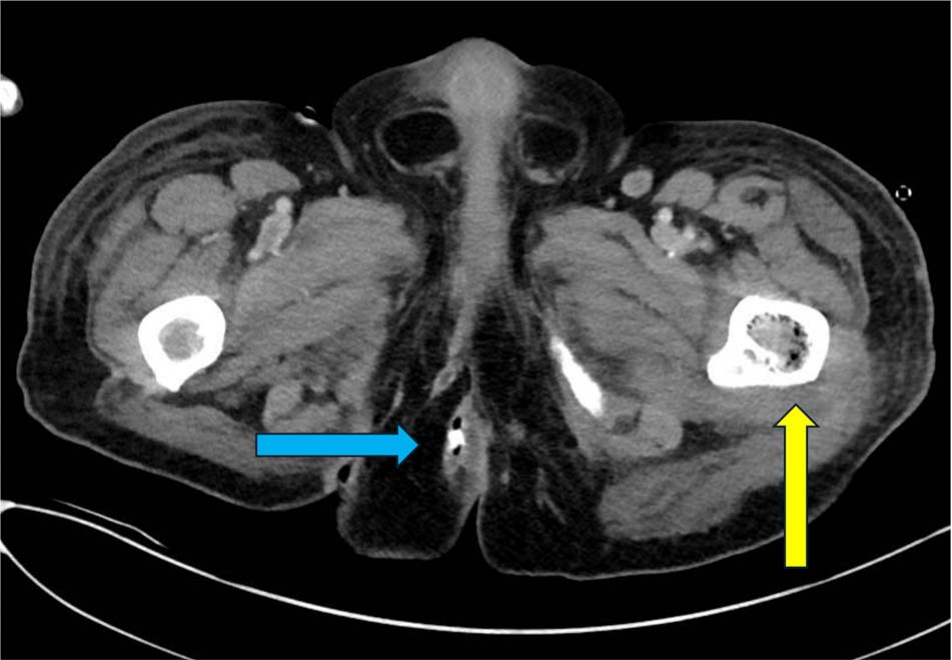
CT abdomen and pelvis—axial plane. Intraosseous gas is seen in the left femur at roughly the level of the lesser trochanter (yellow arrow, right). A previously drained perianal abscess (blue arrow, left).
